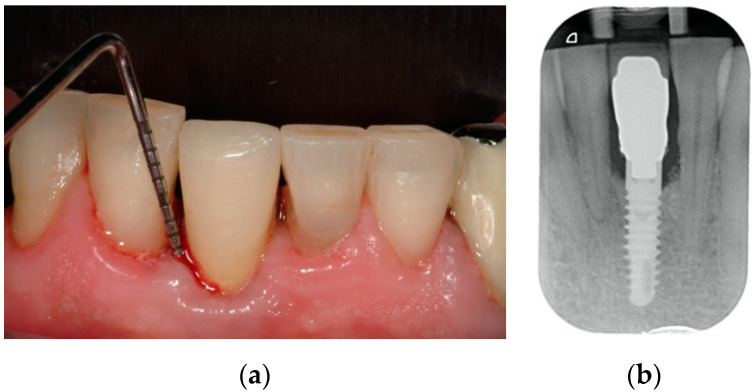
Peri-implant mucositis site of a lower right central incisor: (a) sign of bleeding on probing, and (b) radiographical evaluation showing no bone loss around implant collar.
